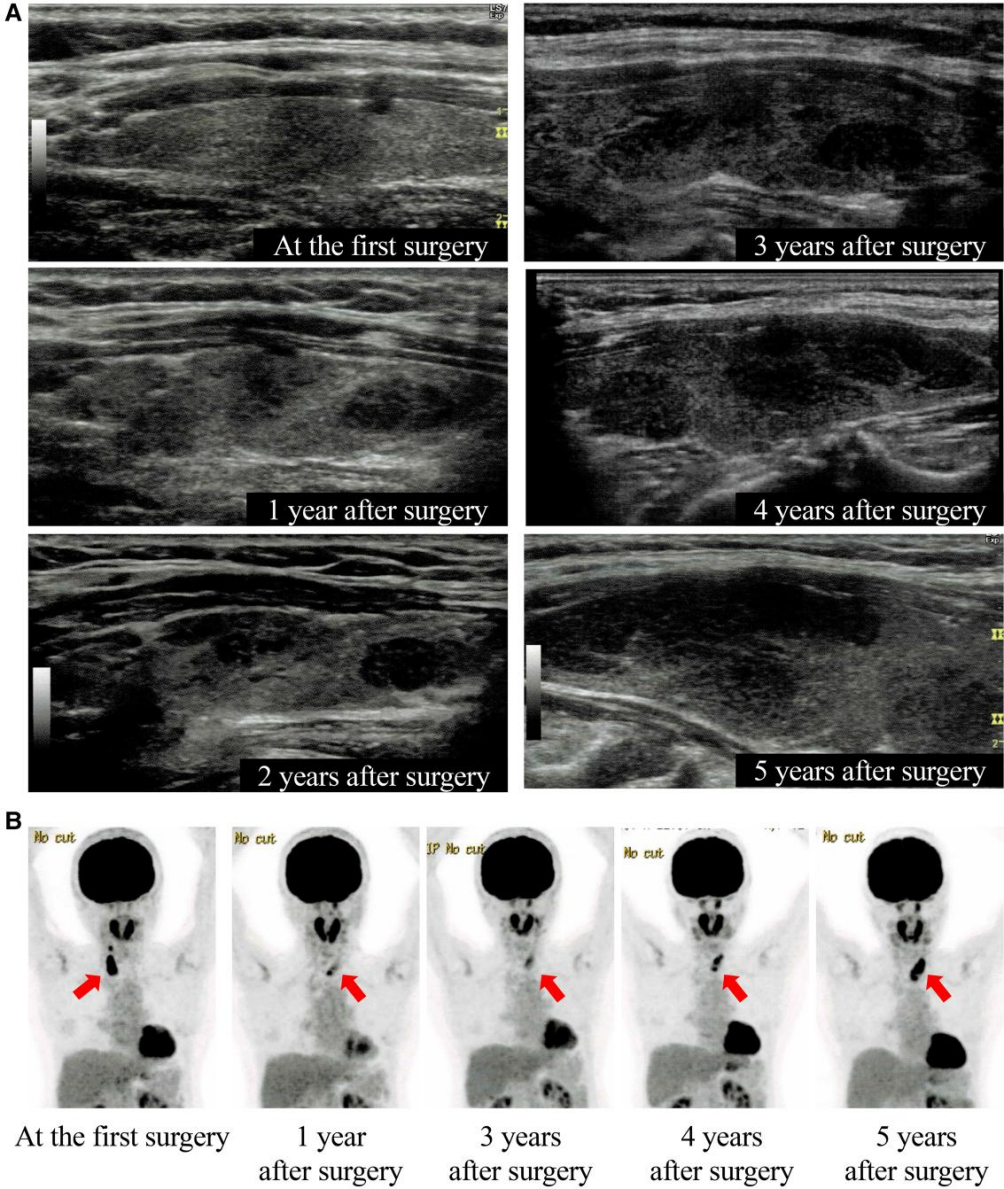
Changes in ultrasound and fluorodeoxyglucose positron emission tomography (FDG-PET) scans following the first surgery. (A) Ultrasound examination showed a hypoechoic area, which appeared in the left lobe 1 year postoperatively. Subsequently, this area enlarged, accompanied by heterogeneous diffuse goiter progression. (B) FDG-PET scan showed a progressive increase in diffuse uptake in the residual left lobe.
